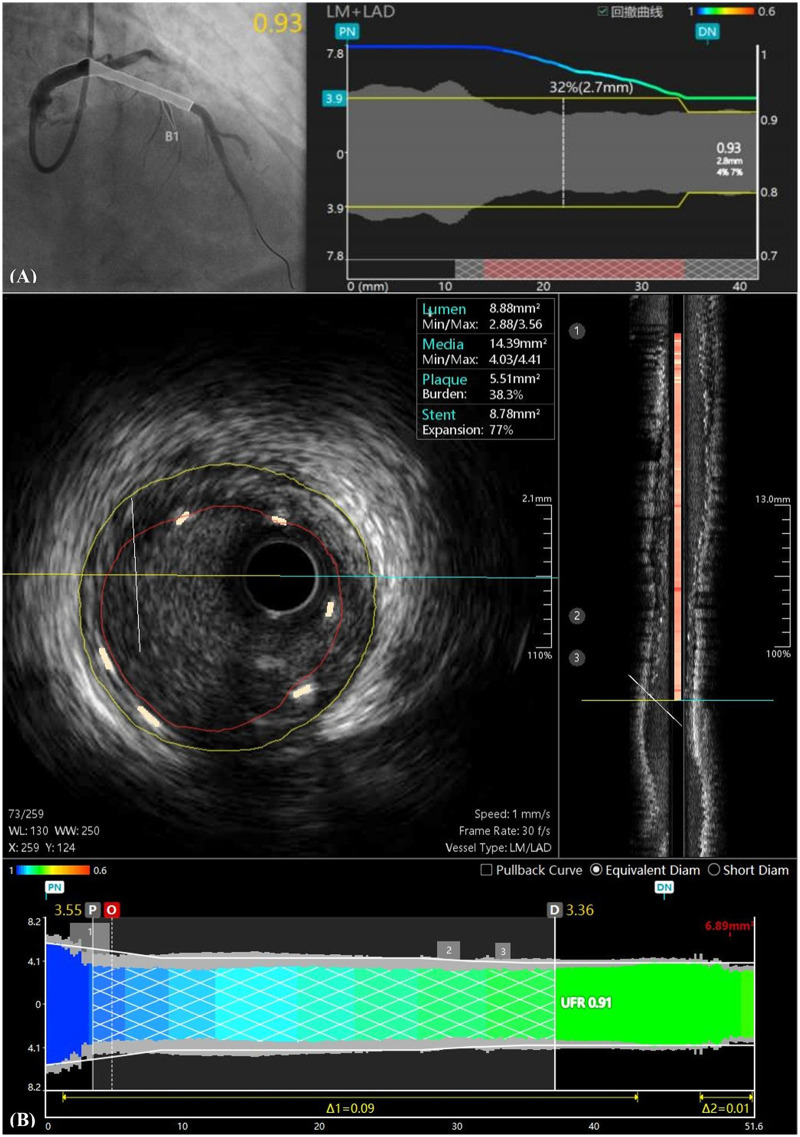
Representative example of post-PCI μQFR and UFR measurements. (A) μQFR measured at the distal exit of the most distal stent = 0.93. (B) UFR measured at the distal exit of the most distal stent = 0.91. μQFR, Murray law-based quantitative flow ratio; UFR, ultrasonic flow ratio.
